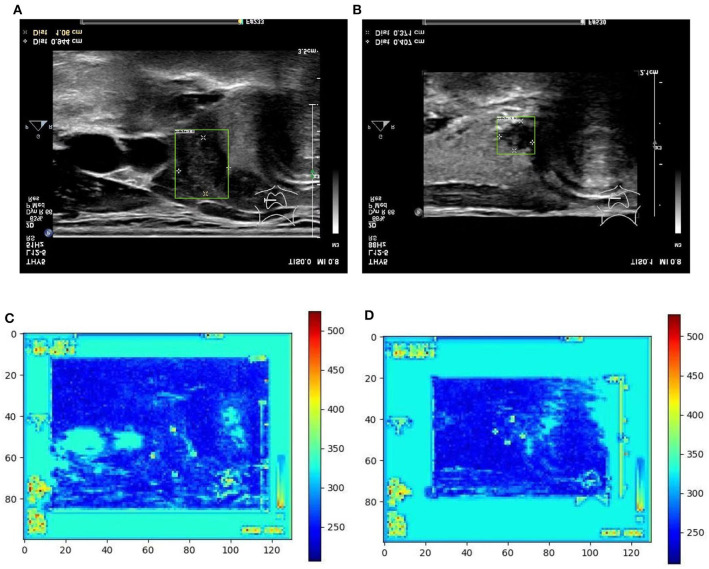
Two examples (from left to right, correspond to the first two-rows in the content of Table 1) of nodule areas which are marker-layered as detected by the bounding box in the ultrasound thyroid image, and their corresponding response heat map as visualized by the feature map in the detection part of the networks. Note the heat maps are computed on the model trained by samples whose markers are kept. Best viewed in color. (A) Nodule in ultrasound thyroid image. (B) Nodule in ultrasound thyroid image. (C) Corresponding response heat map. (D) Corresponding response heat map.
